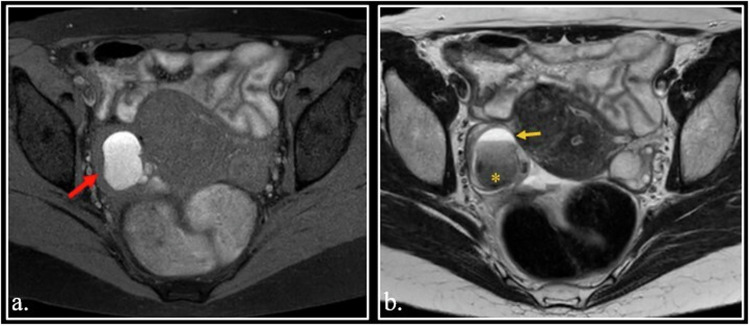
Axial FSE unenhanced T1-weighted fat-sat image (a) shows a homogeneously hyperintense endometrioma (red arrow) of the right ovary. On Axial FSE T2-weighted image (b), the lesion is hypointense (asterisk) (shading sign) with a thin layer of hyperintensity in the upper part of the cyst (orange arrow) due to lower concentration of T1-shortening substances
